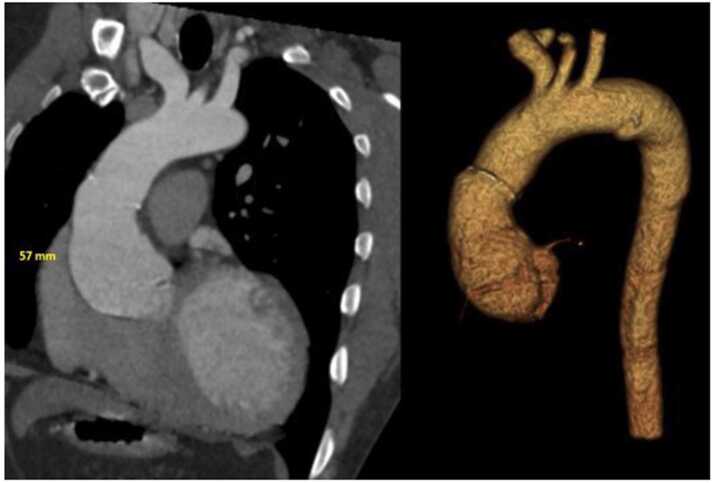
Preoperative aortic computed tomography angiography showing the dilation of the ascending aorta limited to the donor segment of the aorta (not crossing the suture line).
